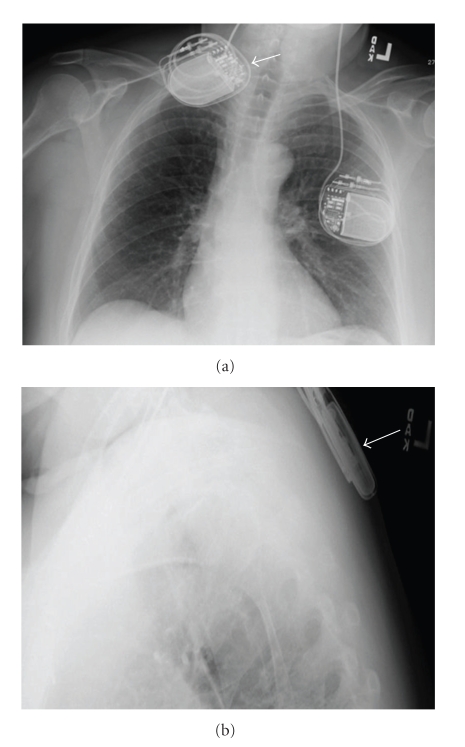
Postoperative X-ray showing placement of the right IPG in suprascapular region after the last surgery.
